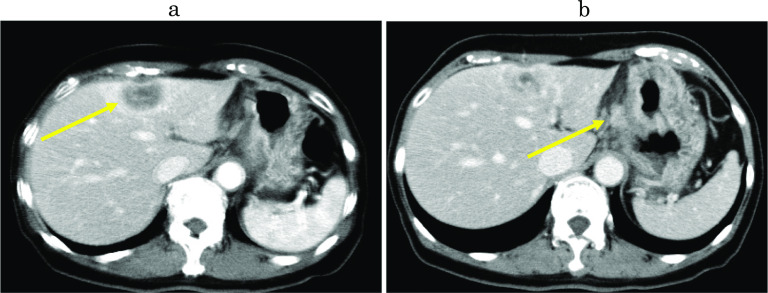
Preoperative abdominal CT imaging. a Enhanced CT showed an irregular enhanced nodule in the left lobe of the liver (yellow arrow). b The enlarged lymph nodes along the lesser curvature of the stomach were detected (yellow arrow)
